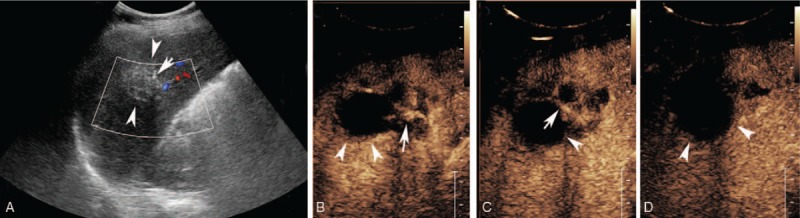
A 46-year-old woman with hepatic AE. A: One nodule (arrow head) with dotted calcifications (arrow) was found in the right hepatic lobe. No signal was detected using color Doppler mode. B: CEUS showed rim enhancement (arrow head) and hyper-enhanced internal septa (arrow) with irregular unenhanced areas in the arterial phase. C and D: In the portal phase and late phase, contrast agent wash-out was seen at the rim (arrow head) and the enhanced septa (arrow), and the un-enhanced area remained. AE = alveolar echinococcosis, CEUS = contrast enhanced ultrasound.
